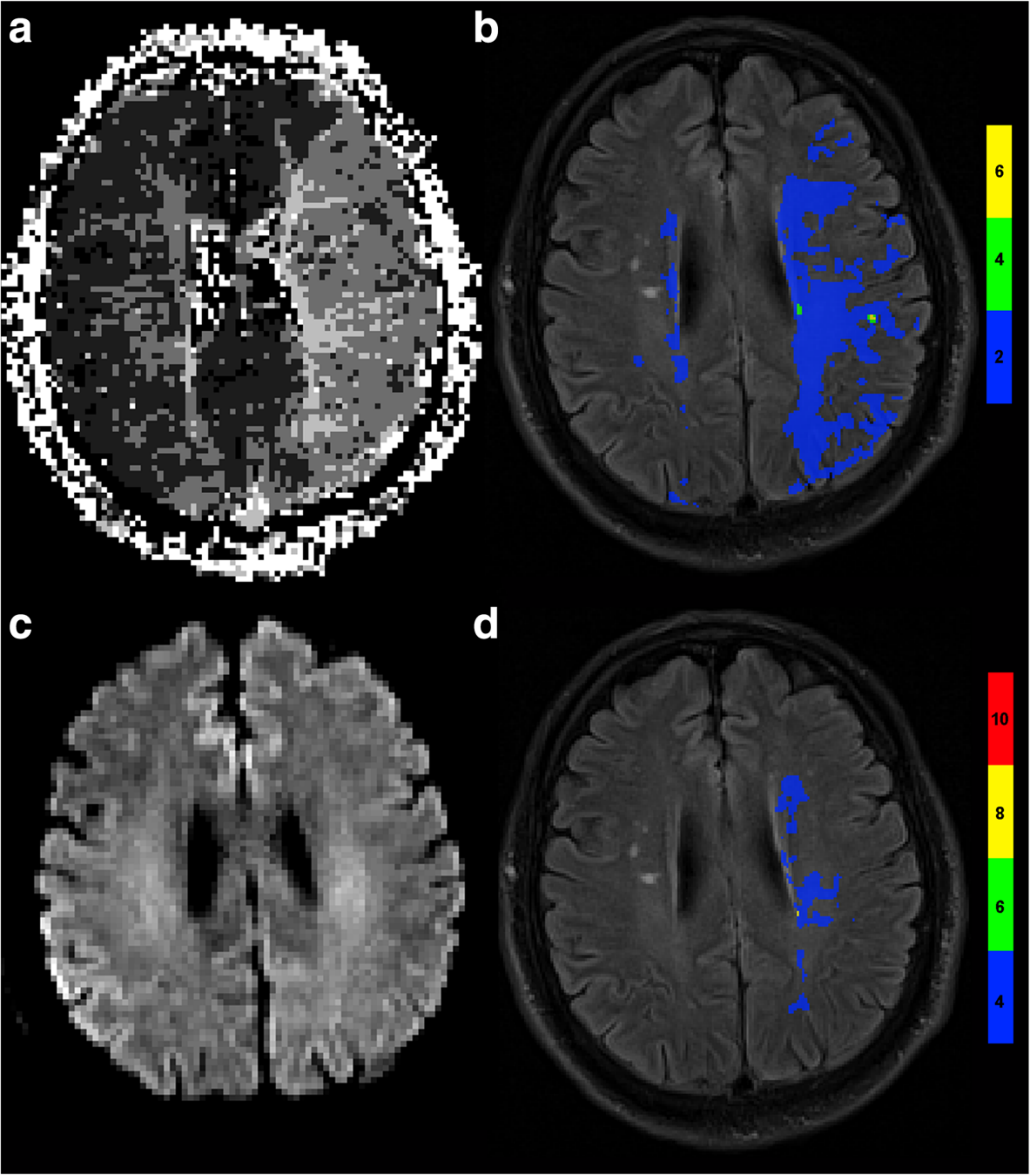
Patient with 90 % carotid artery stenosis and favorable outcome. a Large prolonged TTP volume on TTP-map provided by scanner software without thresholding. b Slight prolongation of TTP volume on TTP-map calculated by Olea-Sphere®. Maps were visualized on fluid attenuated weighted images (FLAIR). c DWI provided by scanner software showing no restrictions. d Small prolonged T_max_ volume calculated by Olea-Sphere®
